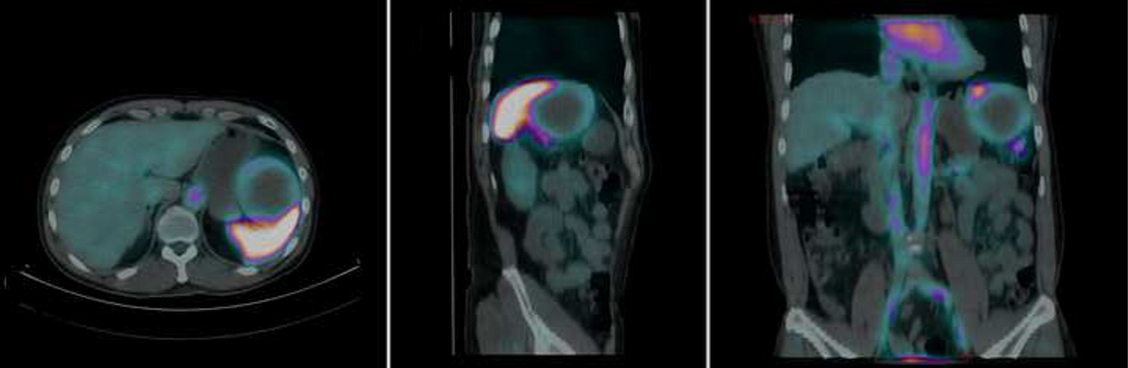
^99m^Tc-RBC scan SPECT/CT fusion images, transaxial, sagittal and coronal of the splenic mass.
